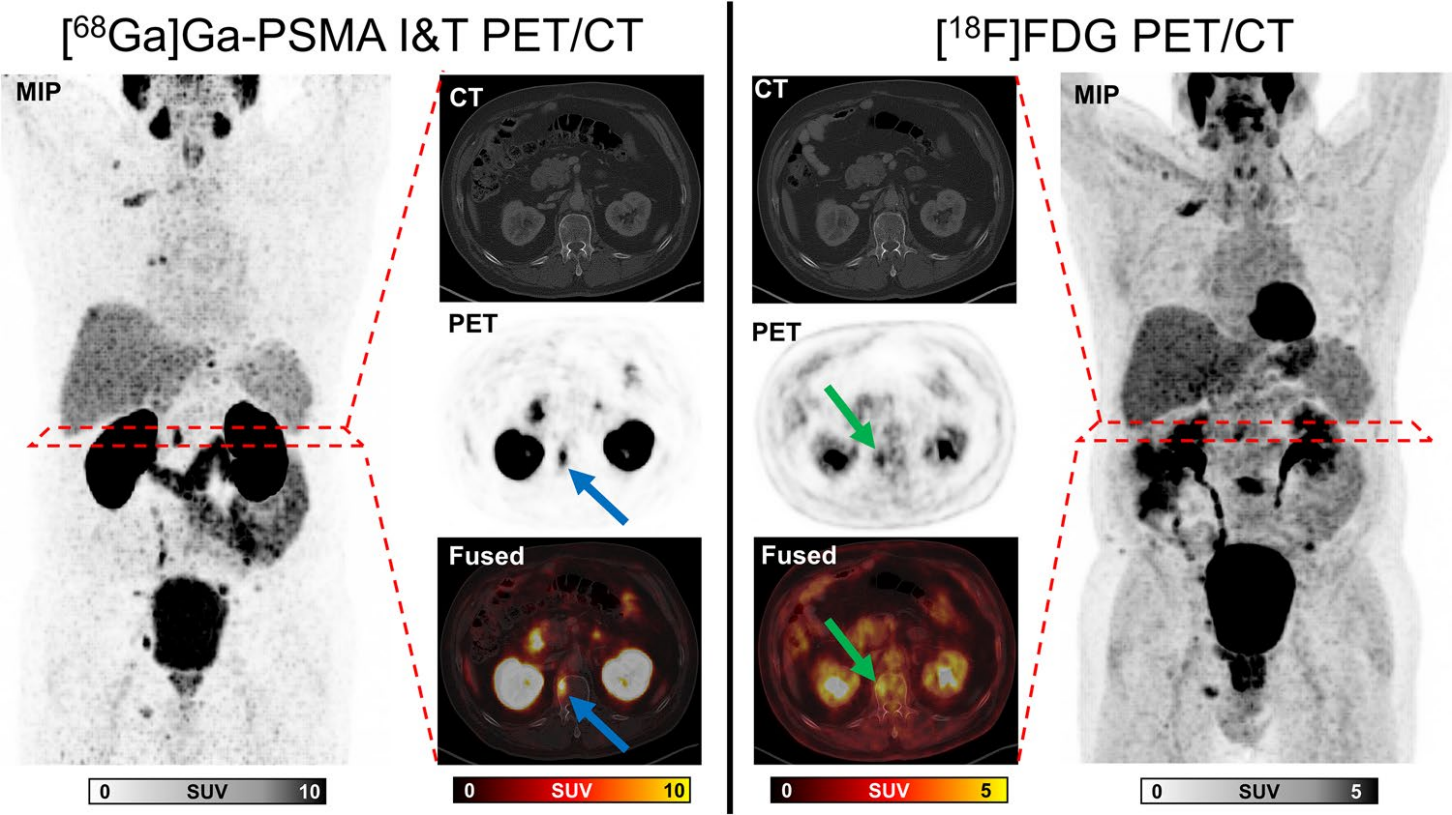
Maximum intensity projections (MIP), CT, PET, and fused images of a man eligible according to VISION criteria (VISION+) but excluded due to TheraP criteria (TheraP−). MIP of [^68^Ga]Ga-PSMA I&T PET (left column) demonstrated multiple lymph node and bone metastases. Highest maximum standardized uptake value (SUV_max_) on [^68^Ga]Ga-PSMA I&T PET was found in the first lumbal vertebral body (blue arrows; SUV_max_ 17.5). The metastasis showed a high uptake (green arrow; SUV_max_ 6.3) on FDG PET (right column). No FDG-positive/PSMA-negative lesions were detected. The patient did not meet the TheraP criteria because no tumor lesions had a SUV_max_ ≥ 20. The patient survived 12 months after initiation of PSMA radioligand therapy
